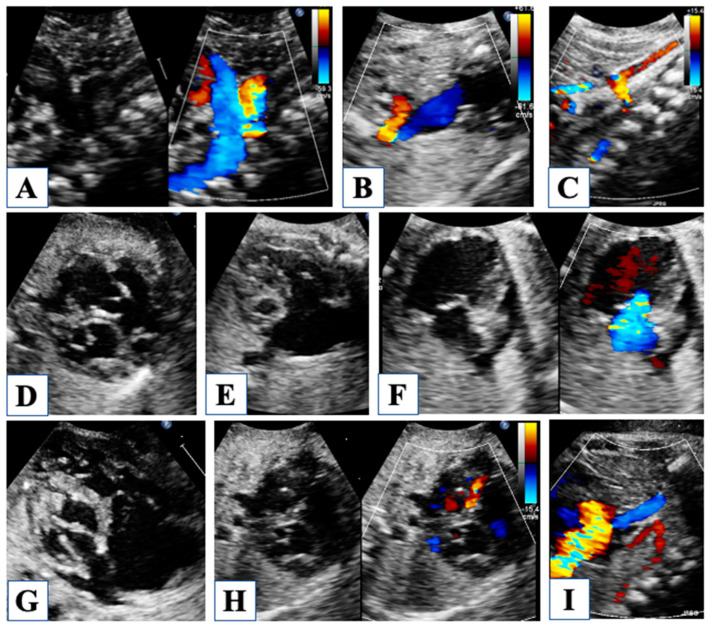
Fetal echocardiography. Reverse flow in the aortic arch is shown from a long-axis view of the aortic arch and ductal arch (panel (A)) and from a three-vessel view (panel (B)). Panel (C) shows severe hypoplasia of all the segments of the aortic arch with retrograde flow. Four-chamber view in panel (D) shows mitral valve stenosis with hypoplastic left ventricle, which does not form cardiac apex. In the four-chamber view in panel (E) mitral valve is atretic, and the left ventricle is a virtual chamber. Tricuspid valve with thickened leaflets and severe regurgitation is shown in Panel (F). In panel (G), the mitral valve is highly dysplastic, with a very small opening in diastole. Panel (H) shows a left-to-right shunt through the foramen ovale with inverted movement of the membrane. A vertical vein draining into the left atrium is shown in Panel (I).
